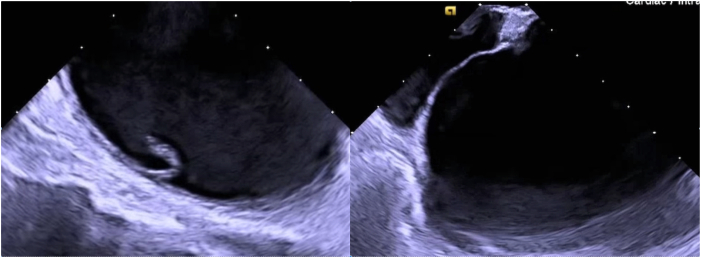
Endothelial disruption of the left atrium after pulsed field ablation. The lesion is clearly visible with intracardiac echocardiography placed inside the left atrium (left), but not clearly when images were obtained from the right atrium (right).
